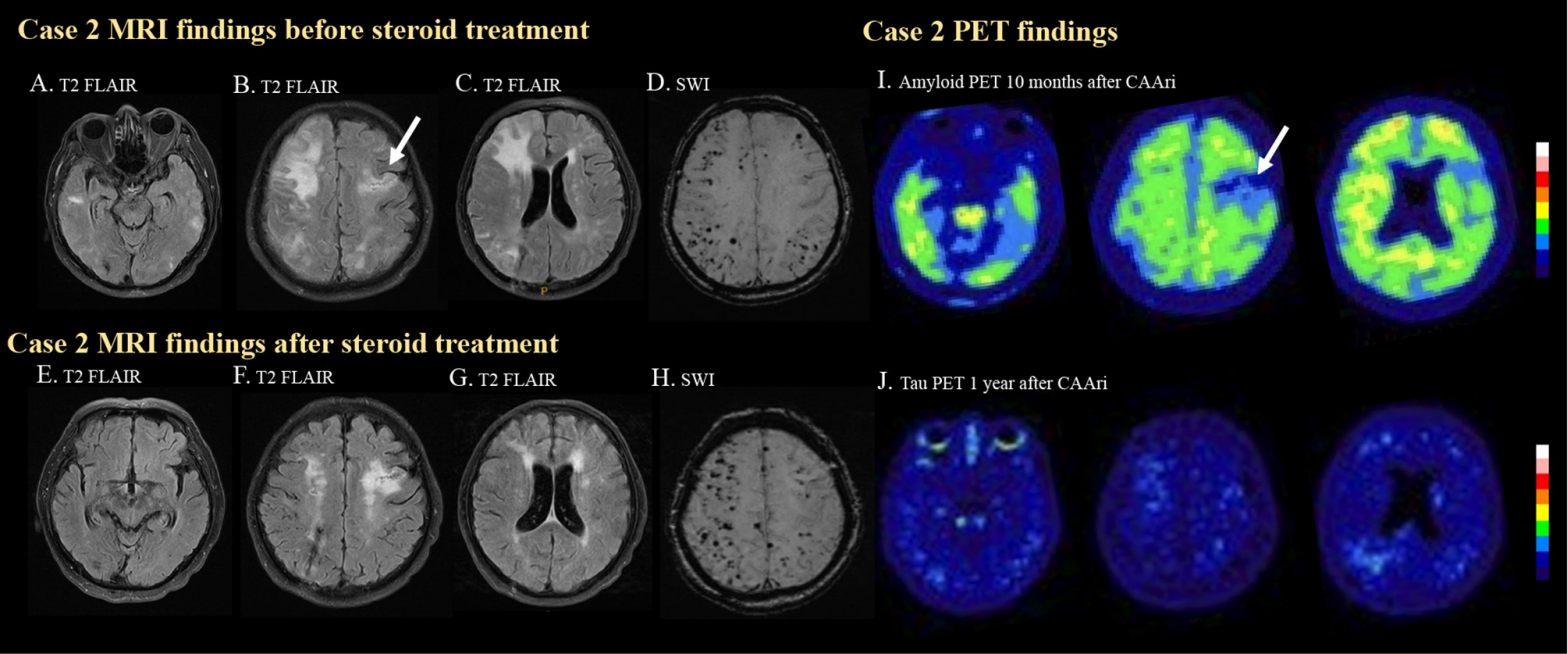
MRI and PET images for case 2. In the second case, multiple hyperintense foci in the bilateral temporal lobes (A) and cortical and subcortical edema in the right frontal and bilateral parietal regions (B, C) were observed on T2 FLAIR imaging before steroid treatment. Previous ischemic insult was also demonstrated [(B), arrow]. Multiple strictly lobar microbleeds were detected in the bilateral cerebral hemispheres on SWI. (D) Follow-up T2 FLAIR imaging after steroid treatment showed resolution of the aforementioned edematous changes and hyperintense foci (E–G). The microbleed became more prominent after the CAA-ri (H). Amyloid PET confirmed amyloid deposition at 10 months after CAA-ri, but the uptake pattern did not correlate with the regions of FLAIR hyperintensities (I). Tracer binding was not observed in the regions affected by previous ischemic insults [(I), arrow]. No significant tau deposition was observed on a tau PET scan (J).
